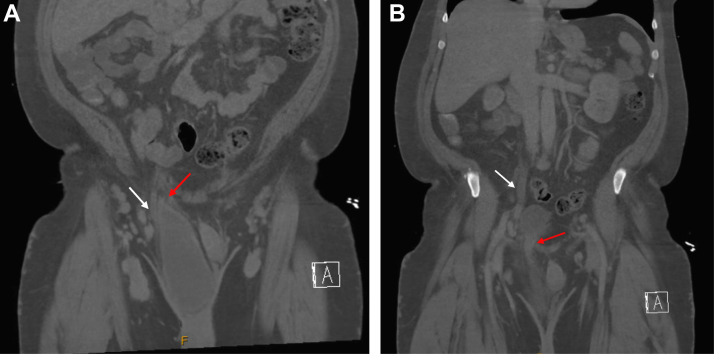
A and B, Computed tomography imaging of the abdomen and pelvis demonstrating ureteral and bladder herniation into an inguinal hernia sac. The white arrow denotes the ureter, and red arrow denotes the bladder as it passes into the hernia sac.
